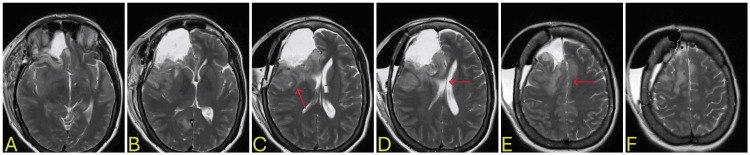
Day 4 brain MRI after lesion resection and lobectomy and prior to discharge A-F: postoperative changes in the right frontal region, D-E: area of swelling and midline shift (red arrow) MRI: magnetic resonance imaging
